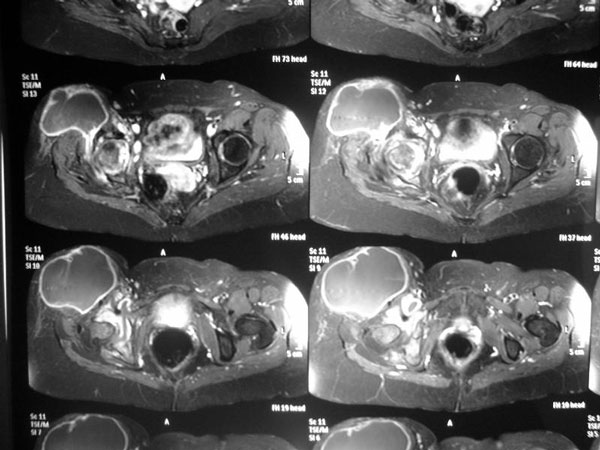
Magnetic resonance imaging of the hip showing a large periarticular abscess and destruction of the right femour head.
